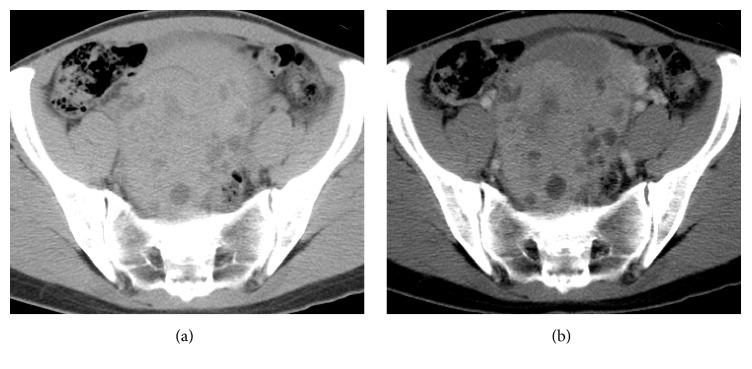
(a) Plain axial computed tomography (CT) depicting a mass of approximately 12 × 9 cm with heterogeneous density. (b) Contrast-enhanced axial CT showing the cystic area of the enhanced solid mass.
